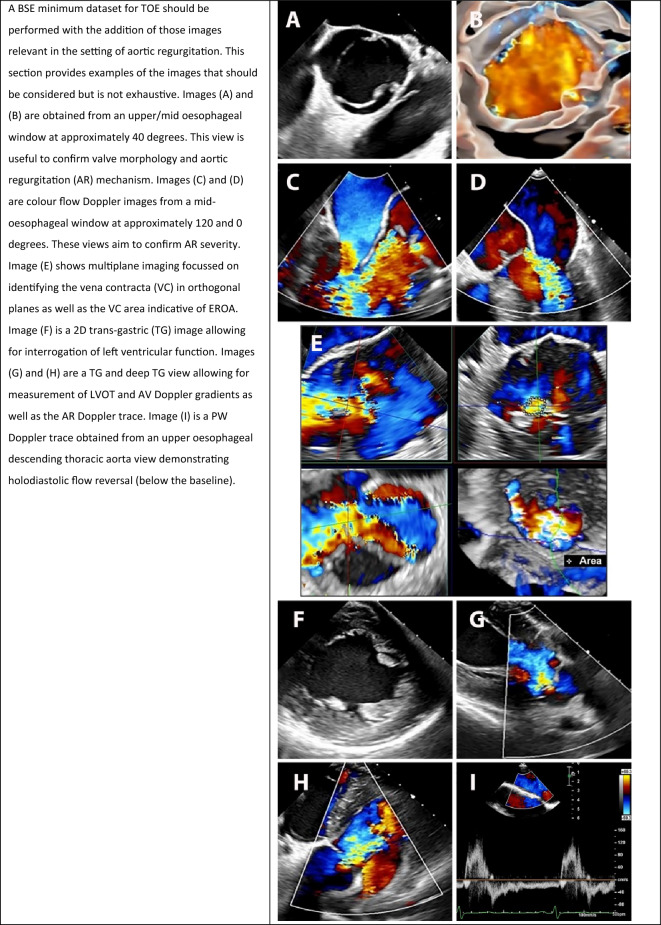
Transoesophageal echocardiographic (TOE) images which complement transthoracic echocardiography
